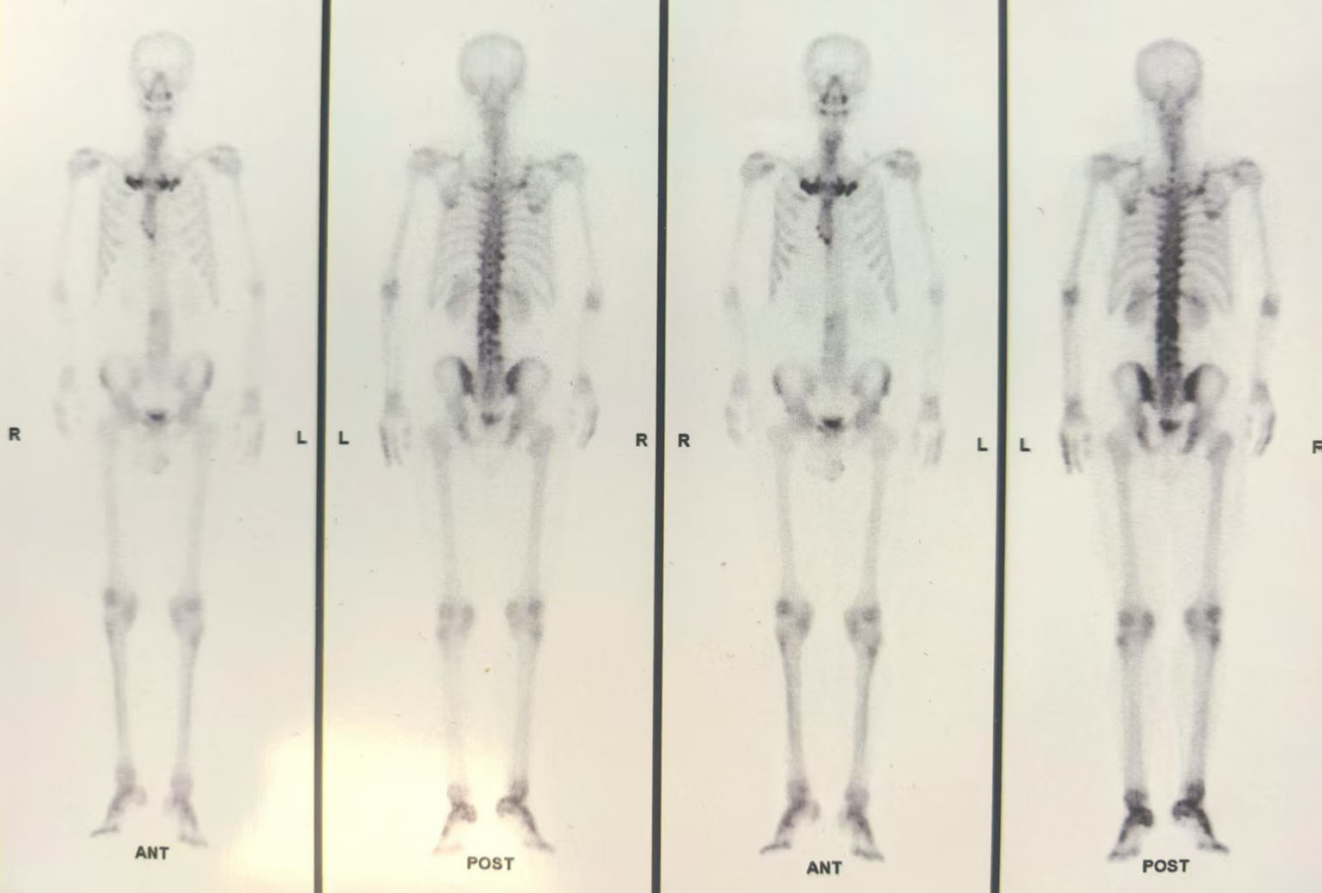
Bone scintigraphy. Pre-treatment increased radioactive uptake (tomographic slice thickness: 5 mm). Bone scan findings before treatment indicated typical “bull head sign”, osteomyelitis and Synovitis.
